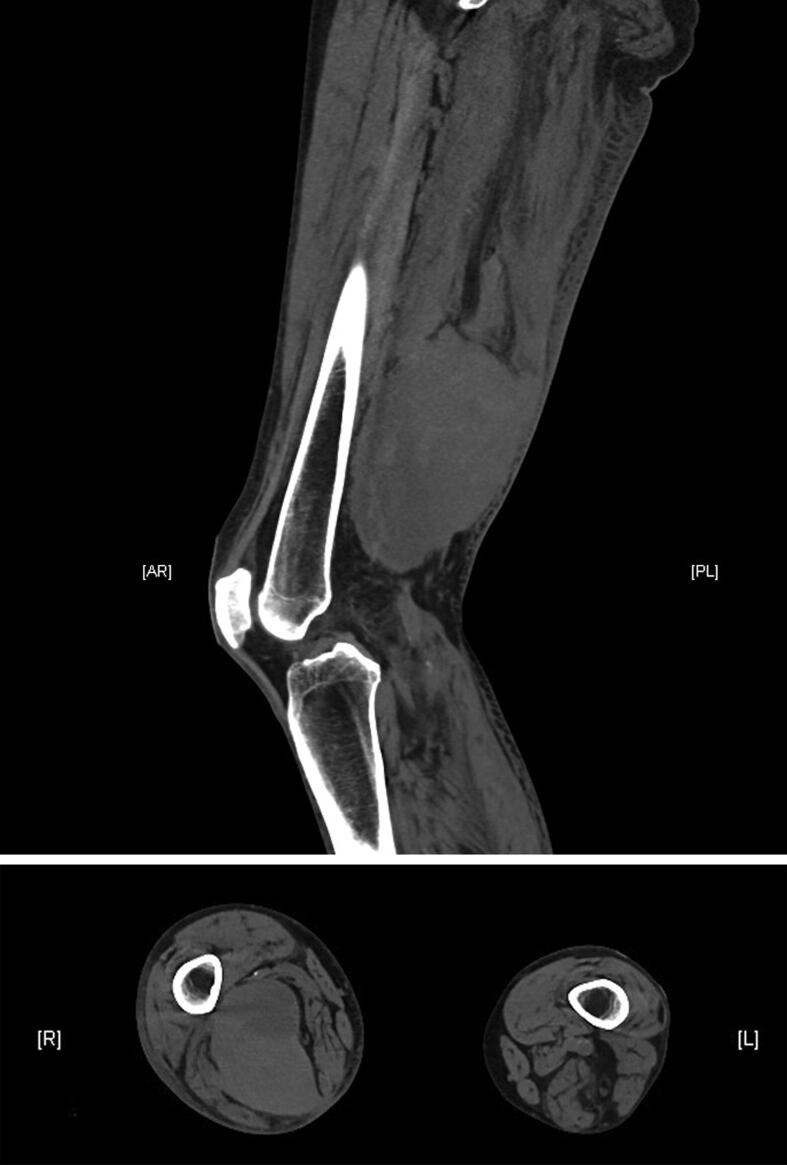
Computed tomography (CT) image of a) a partially intramuscular hematoma located in the dorsal thigh (15 × 10 × 7,5cm) and b) compressing the sciatic nerve (Patient 2).
